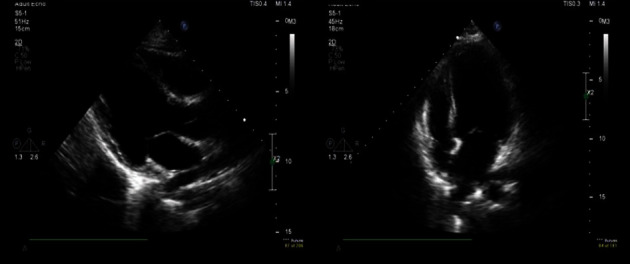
Echocardiogram postcardiac arrest and resurrection. Apical view and long axis parasternal view.
